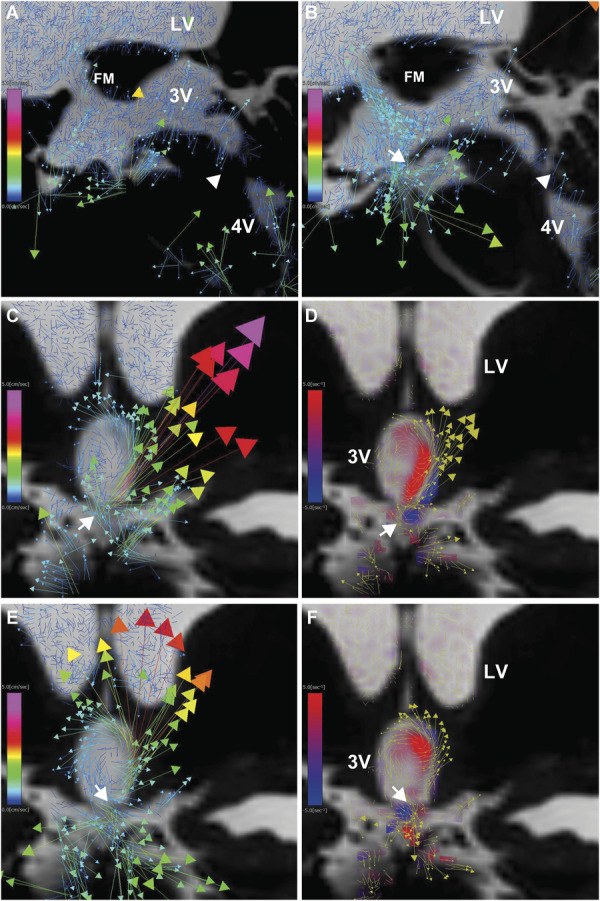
Obstructive hydrocephalus (case 2). A, Sagittal view of 4D flow MRI before treatment. The white arrowhead indicates the obstructed site at the lower end of the cerebral aqueduct, and the colored arrows represent velocity vectors. A slight CSF flow velocity was observed around the FM. B, Sagittal view of 2D velocity mapping on 4D flow MRI after ETV. Rapid CSF flow was observed from the FM into the 3V and through the fenestration in the 3V floor (white arrow) into the interpeduncular cistern. C-F, Coronal views of 4D flow MRI after ETV, showing velocity maps with colored arrows indicating velocity vectors C and E, and vorticity maps D and F. The vorticity maps show clockwise vortices in blue, counterclockwise vortices in red, and overlaid yellow velocity vectors. CSF entered the 3V through the fenestration in the 3V floor (white arrow), created a strong counterclockwise vortex, and then exited into the interpeduncular cistern. 2D, 2-dimensional; 3V, third ventricle; 4D, 4-dimensional; 4V, fourth ventricle; CSF, cerebrospinal fluid; ETV, endoscopic third ventriculostomy; FM, foramen of Monro.
